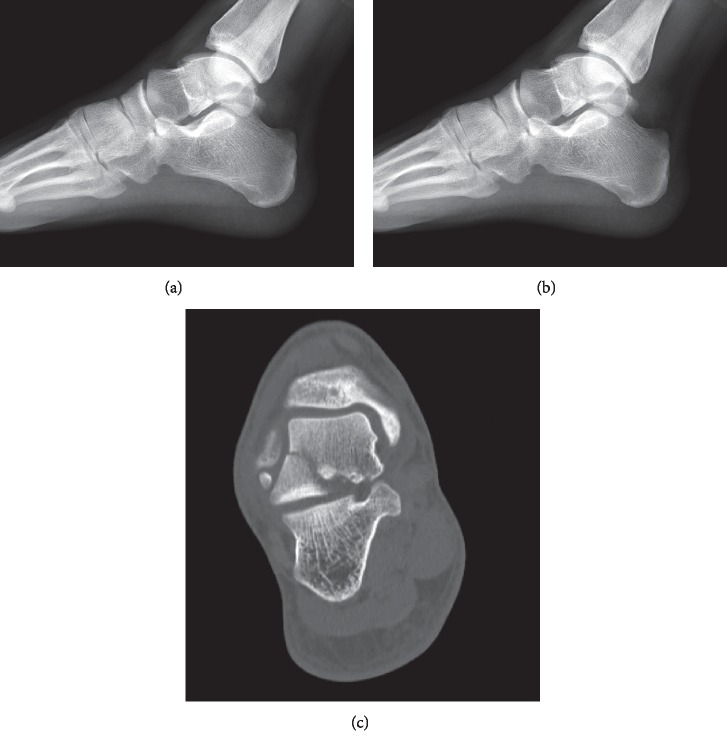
Lateral foot X-ray (a) demonstrating subtle fracture, vertically oriented across the talar body. Sagittal (b) and coronal (CT) cuts better elucidating fracture pattern. Primary fracture line extends posterior to the lateral process of the talus. There is intraarticular extension into the middle and posterior subtalar joints. Fracture extends into the anterior talar dome with articular step-off.
